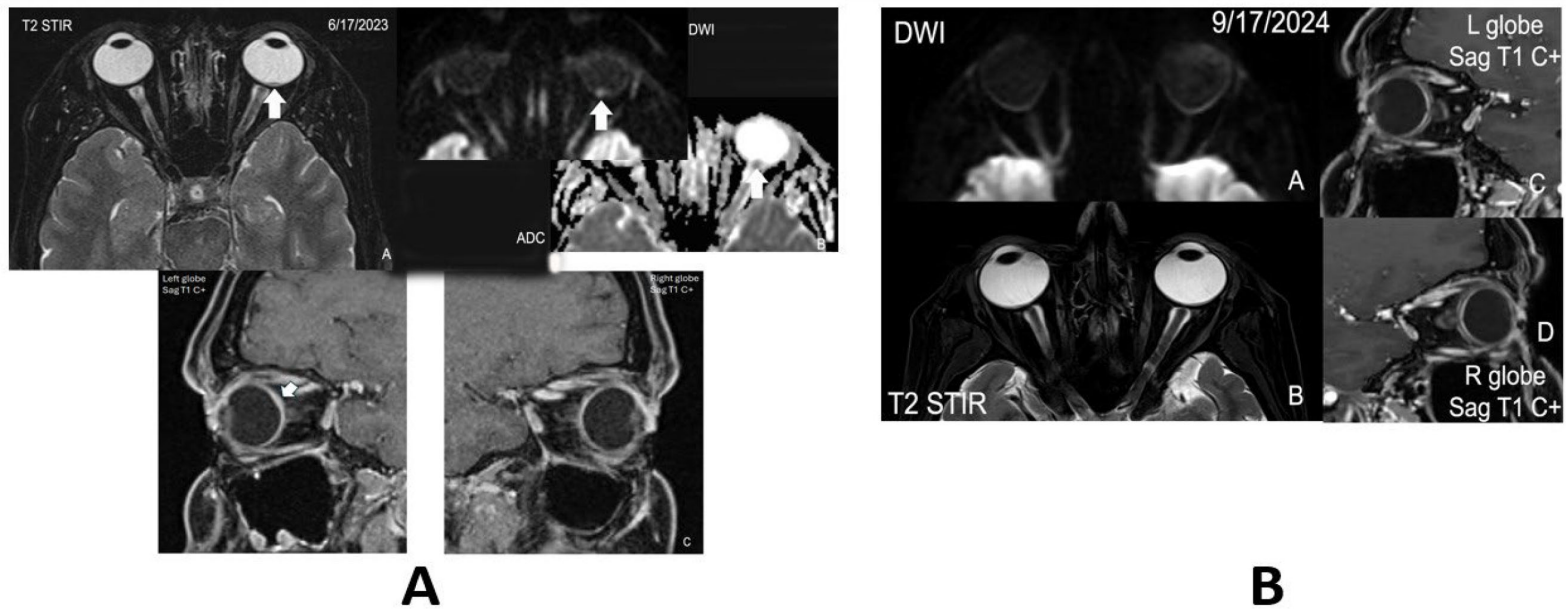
(A) (A-C). Orbit MRI at diagnosis. (A) Orbit MRI axial STIR/T2 fat sat images show a region of focal left choroidal thickening (white arrow). (B) Orbit MRI axial DWI and ADC images demonstrate the lesion near the optic disc with true restricted diffusion indicating cellularity. (C) Sag oblique T1 post-contrast 3D/volumetric VIBE sequences show a very subtle choroidal thickening not seen on any other sequences, more conspicuous compared to the normal right globe/choroid. (B) (A-D). Post-treatment orbit MRI images. Post-treatment orbit MRI with axial DWI (A), T2 STIR images (B) demonstrating resolution of the choroidal lesion with more symmetric uveoscleral layers after resolution of the focal left choroidal thickening seen previously on post-contrast images [white arrow, (C)] compared to the normal right globe (D).
